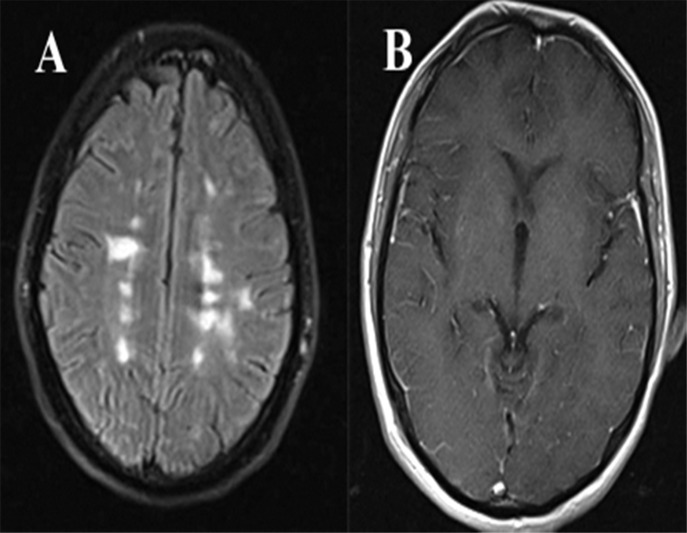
Periventricular lesions confirming the diagnosis of multiple sclerosis (MS) in the FLAIR MRI view (A). Meningeal enhancement was seen after gadolinium injection (B)
